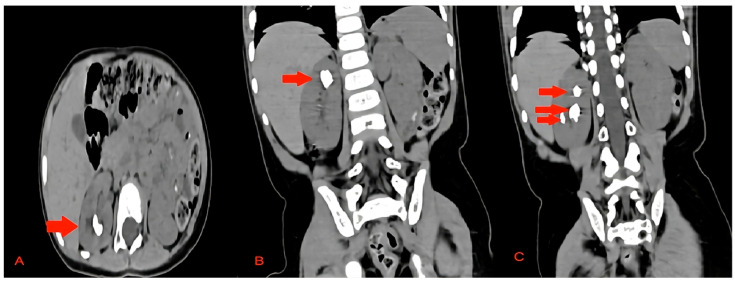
The preoperative CT scan of this case showing the stones in the right kidney. (A) Axial view of the upper pole stone, (B) coronal view of the upper pole stone, and (C) coronal view of the upper pole and middle pole stones. The stones are marked with red arrows.
